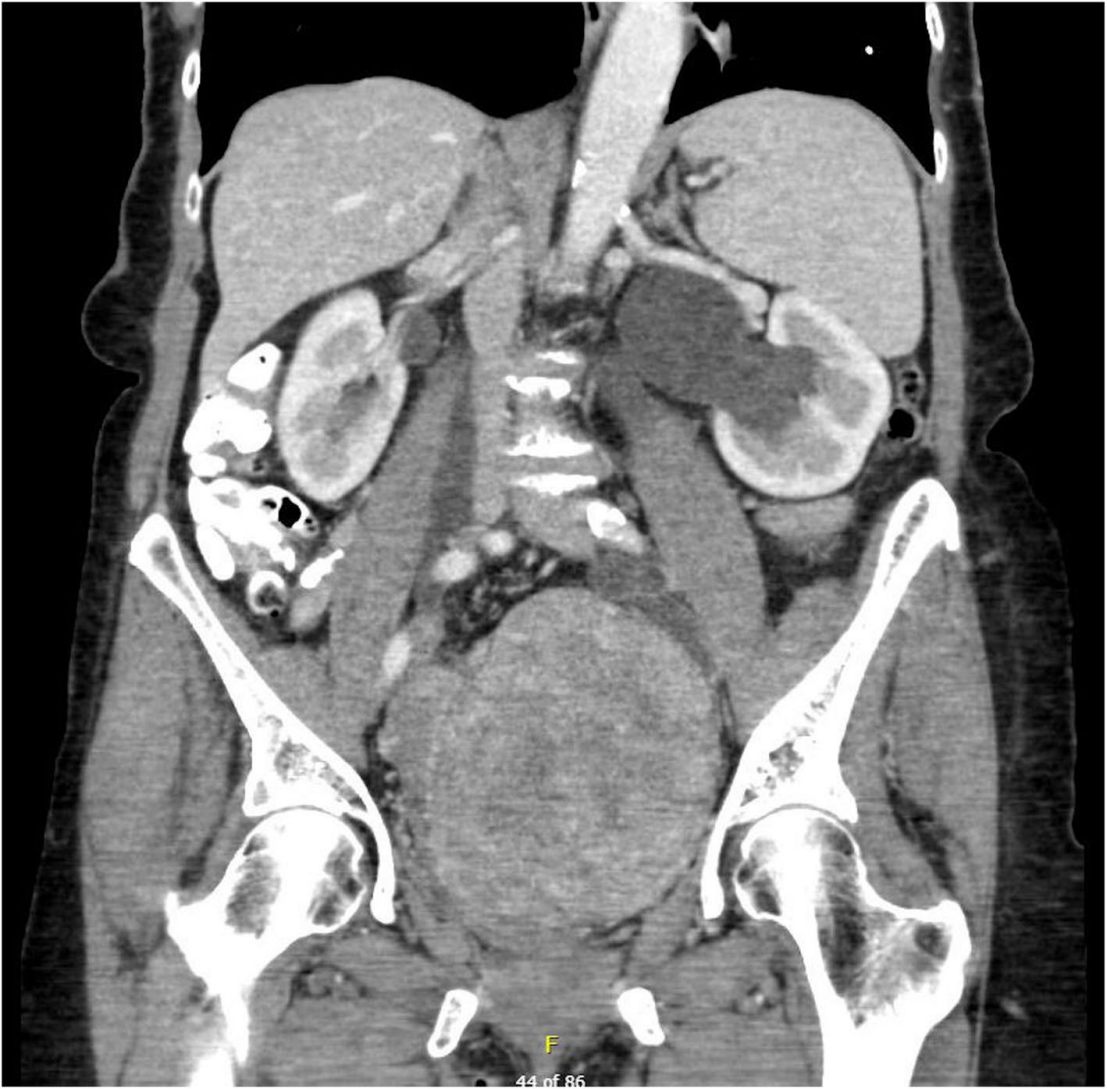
Computed Tomography imaging of pelvic mass
